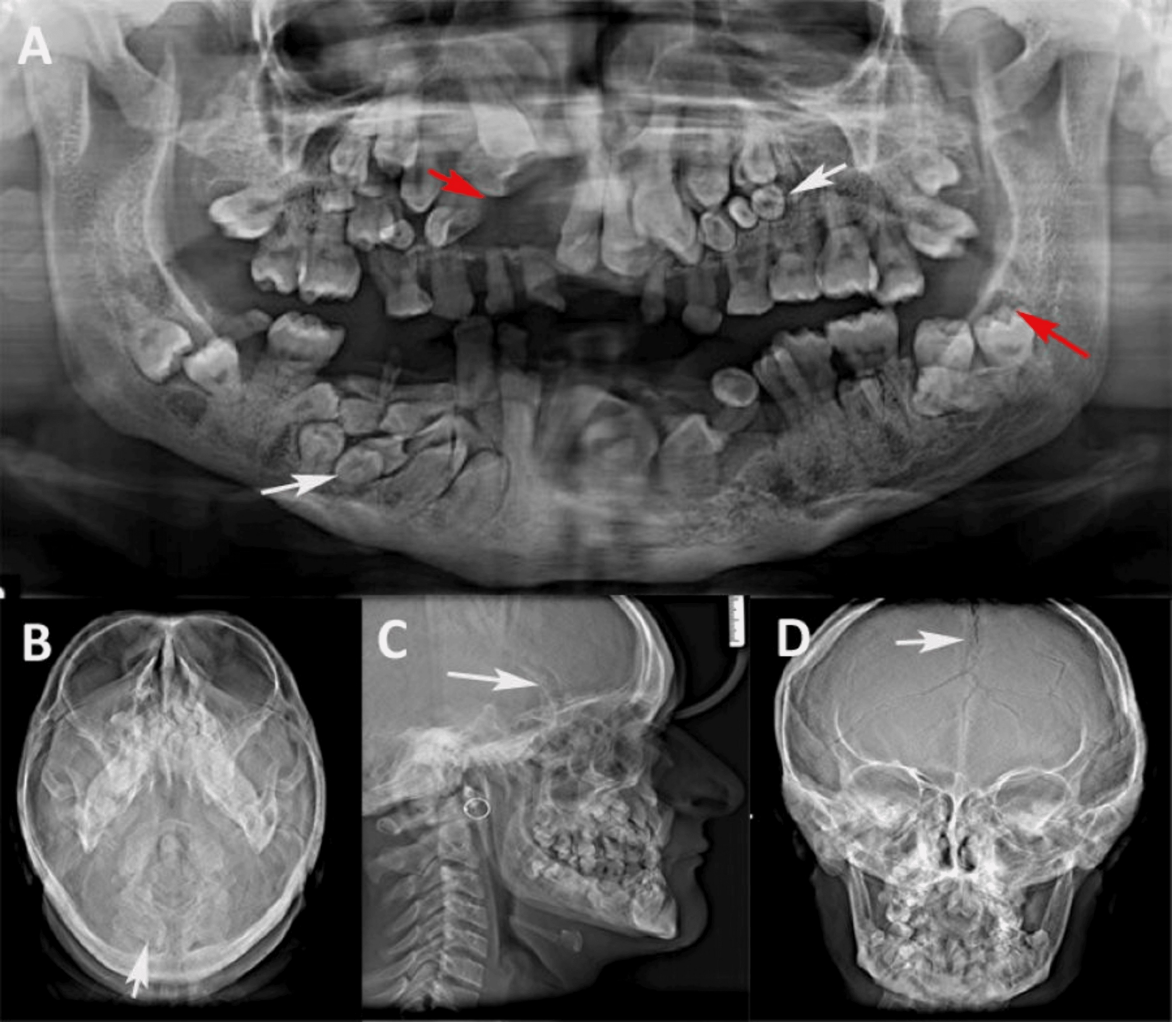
Radiographic features (A) Orthopantomogram (OPG) revealing multiple retained deciduous, impacted permanent, and supernumerary teeth (white arrows), with radiolucency (red arrows) indicating cystic changes. (B) Paranasal sinus (PNS) view showing underdeveloped sinuses and increased sutural markings on the skull. (C) Lateral cephalogram displaying a hypoplastic maxilla, prognathic mandible, numerous impacted teeth, and delayed cranial suture closure (white arrow). (D) Posteroanterior skull radiograph showing open cranial sutures and fontanelles (white arrow)
